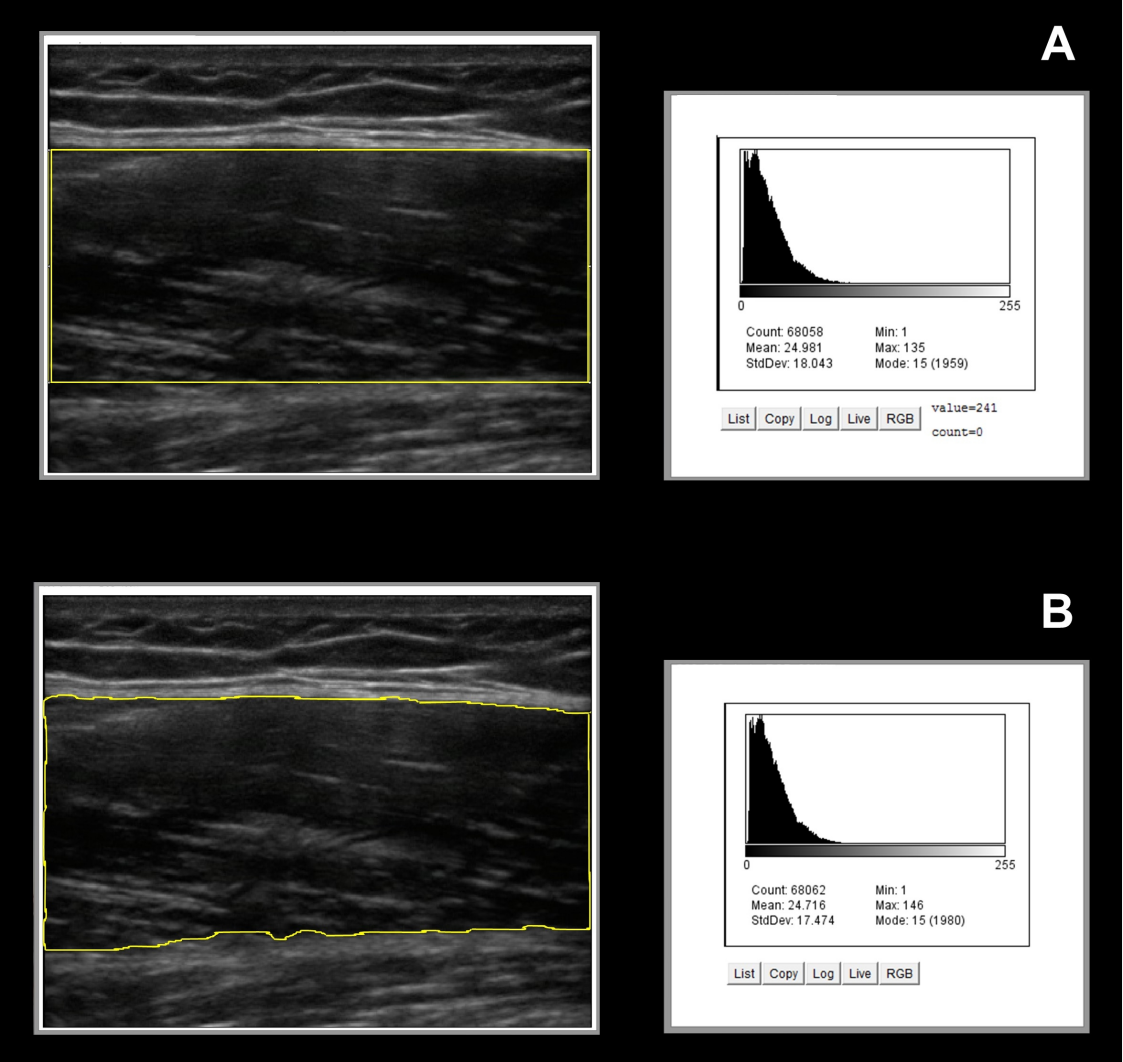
Image analysis ROI selections and the corresponding grayscale histogram values. The exemplar longitudinal images of the rectus femoris feature an overlay graphic of the ROI selection using the Rectangular Marquee Tool (A) and Free Hand Tool (B) provided by the ImageJ software. The corresponding grayscale histogram analysis data for each sonographic image shows similar estimates of rectus femoris echogenicity using both ROI selection methods. (ROI, region of interest. Post-analysis image enhancement used only to emphasize the ROI selection borders in the figure.)
